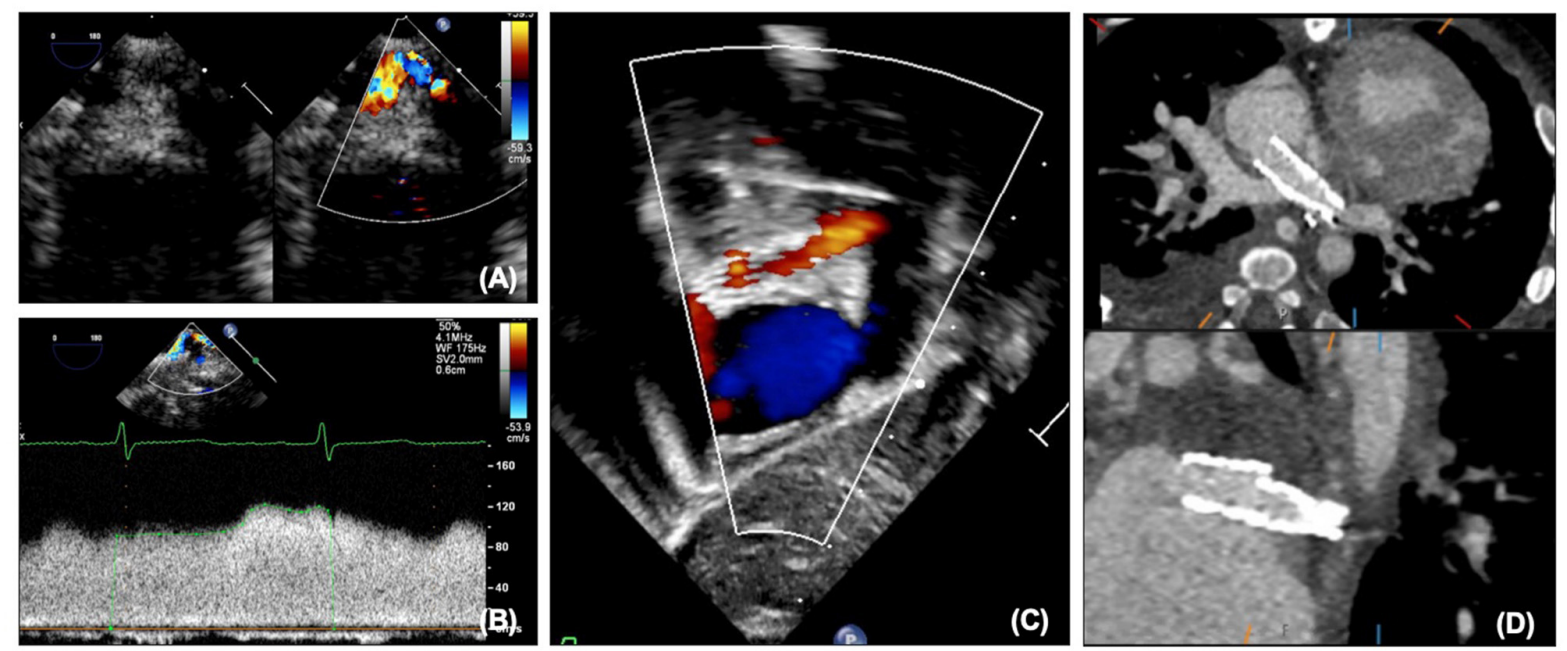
(A) Pulmonary vein stenosis on echocardiography on 2D and color. (B) Abnormal pulmonary vein Doppler due to stenosis. (C) Left pulmonary vein and atrial septal stents on transthoracic echocardiography. (D) Left pulmonary vein and atrial septal stents on CT.
